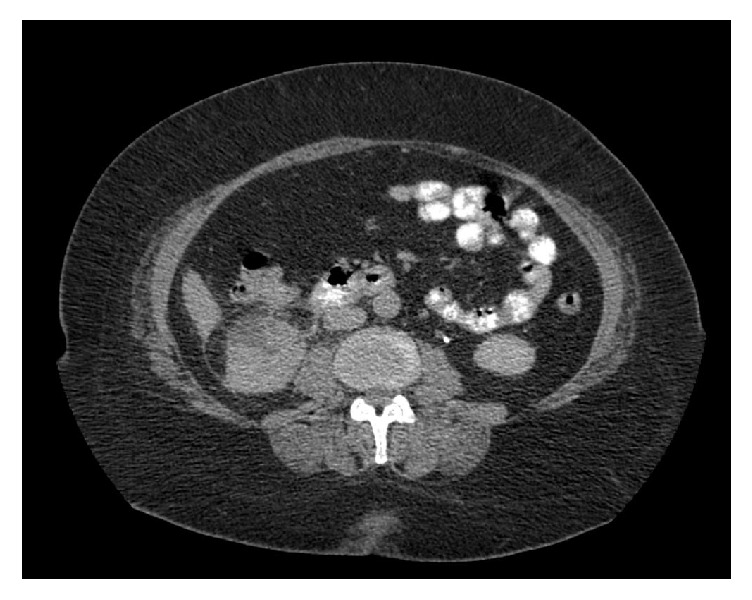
CT demonstrating no signs of contrast leakage from duodenum.
